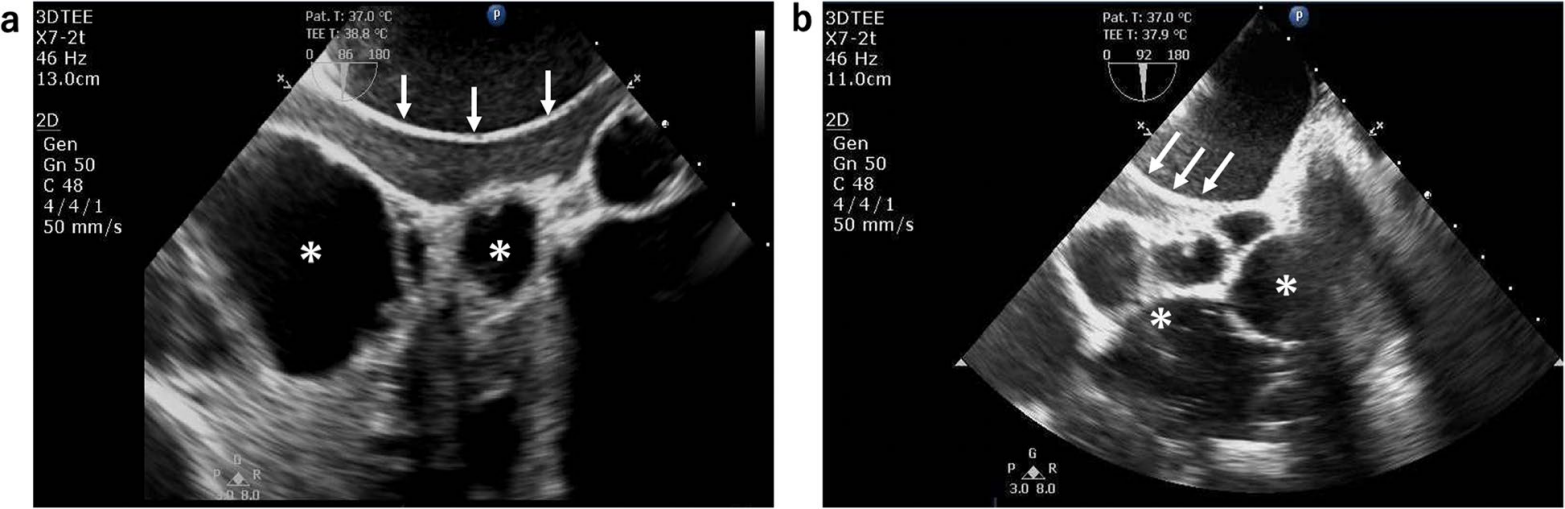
Transesophageal echocardiography (TEE) images of the intrahepatic inferior vena cava (IVC). Before the mechanical liver displacement, the IVC (white arrows) was surrounded by various cysts (asterisks), but was not collapsed (a). TEE indicated IVC collapse (b) due to severe mechanical liver displacement
